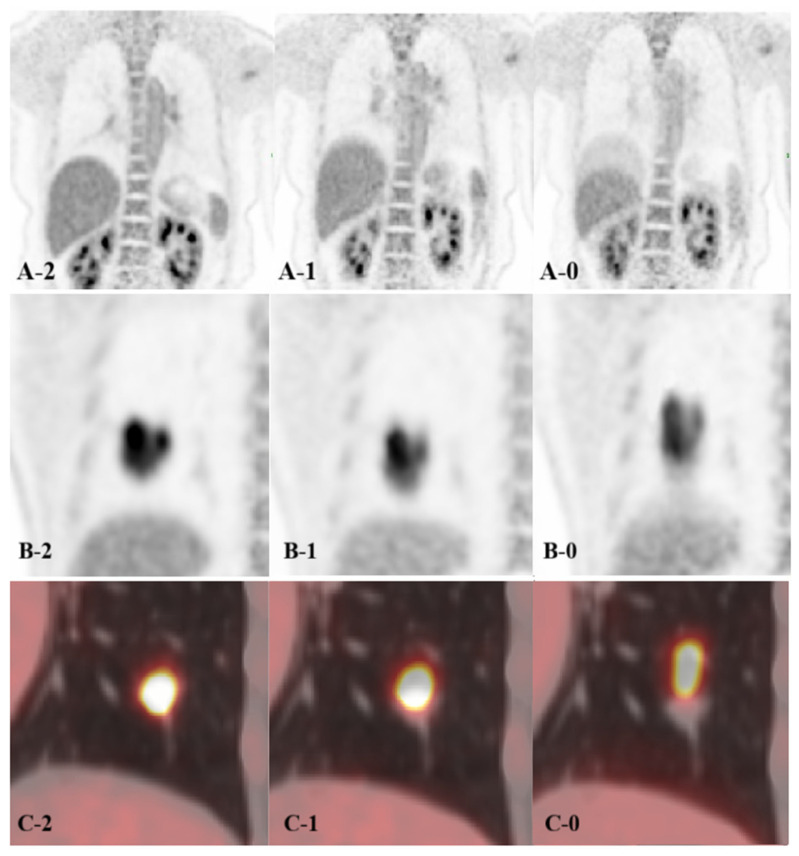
Visual assessment of PET/CT imaging. A: The respiratory motion of the liver; B: lesion clarity; and C: PET/CT alignment. The visual scores: good (2), moderate (1), and poor (0).
